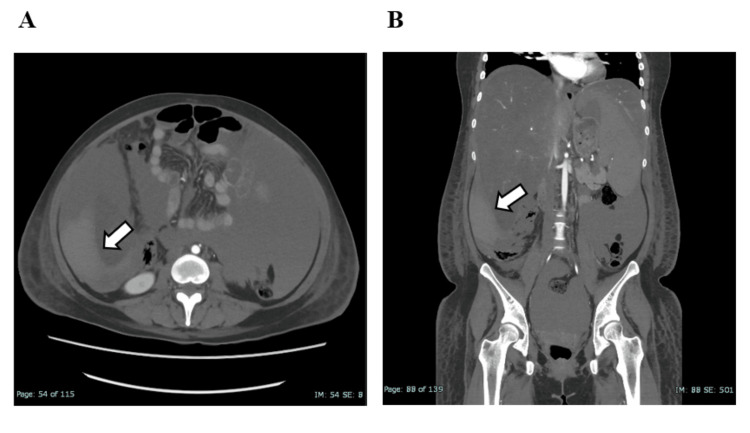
Contrast-enhanced CT after liver biopsy showing hemorrhagic ascites The images show high-density fluid consistent with hemorrhagic ascites surrounding the liver, suggestive of intra-abdominal bleeding. No obvious active extravasation was noted. CT: Computed tomography
